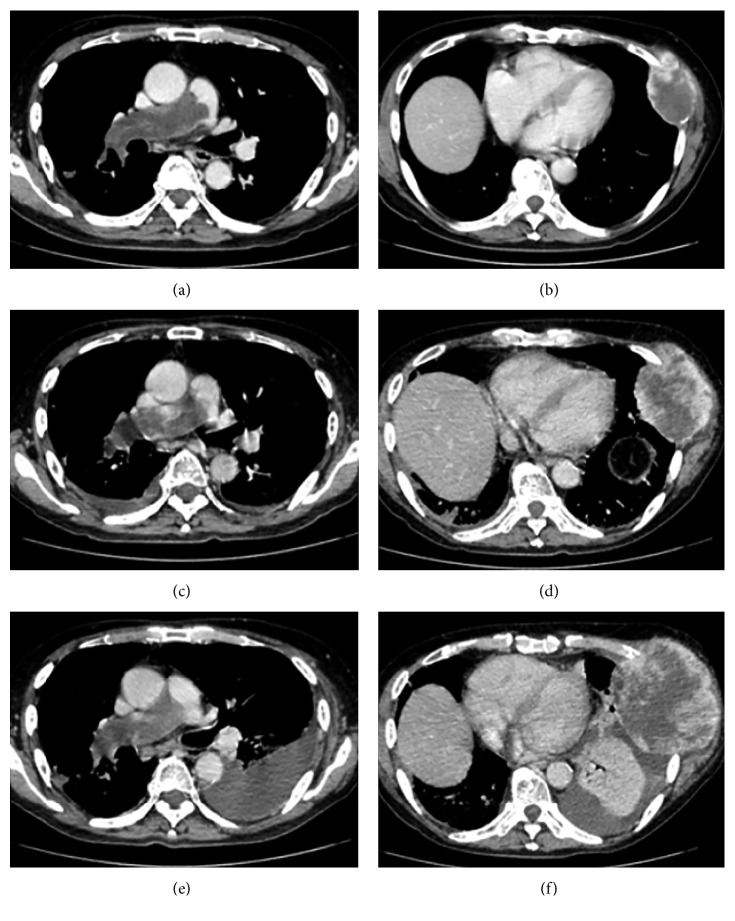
Chronological progression of the tumors on chest computed tomography. Computed tomography cross sections of the right pulmonary artery and chest wall tumor levels were shown at 4 (a, b), 8 (c, d), and 9 months (e, f) from the initial visit. The tumor expansively occupied the entire lumen of the right pulmonary artery, and the chest wall tumor drastically enlarged over time, with a massive left pulmonary effusion appearing at 9 months.
